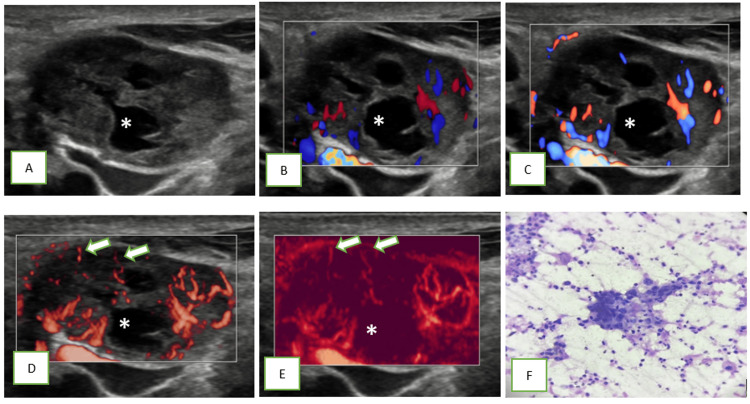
Metastatic deposits of poorly differentiated squamous cell malignancy. A 55-year-old man with a history of biopsy-proven squamous cell carcinoma of the base of the tongue presented with palpable cervical lymph nodes for 7-8 months. (A) Gray-scale ultrasound (US) image showing an enlarged heterogeneous cervical lymph node with central necrotic areas (*) and loss of normal hilar architecture. (B, C) Color Doppler and power Doppler, respectively, show distorted peripheral vascularity with a necrotic area (*). (E, F) MVI (color) and MVI (power) modes, respectively, demonstrated enhanced irregular peripheral neovascularization (white arrows) with distorted microvascular architecture with necrotic areas (*)-likely suggesting metastatic malignant etiology. (F) FNAC shows clusters of atypical pleomorphic malignant squamous cells with hyperchromatic nuclei and keratinous debris, consistent with metastatic deposits of poorly differentiated squamous cell malignancy. MVI: microvascular imaging; FNAC: fine-needle aspiration cytology
